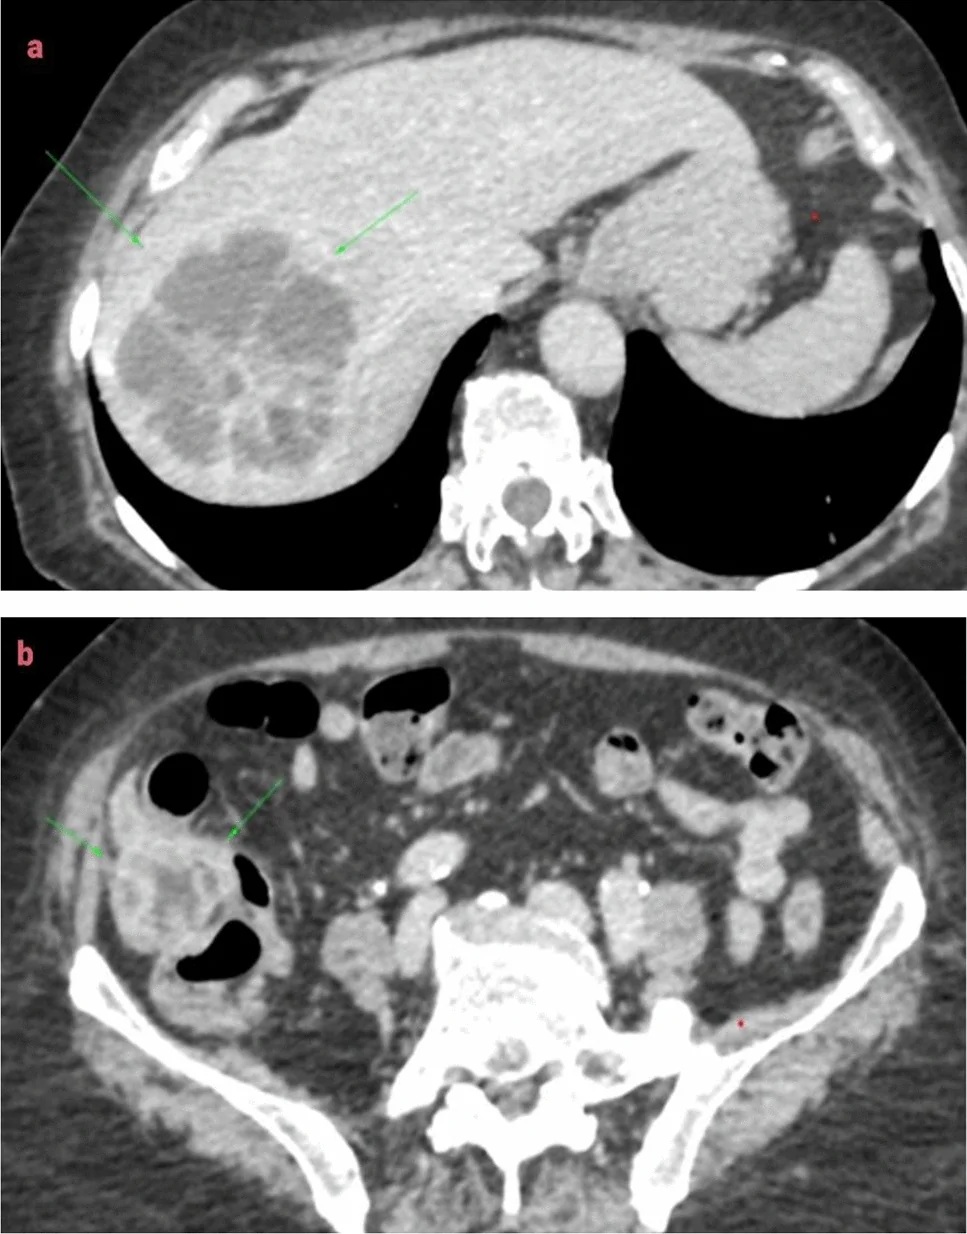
CT image of the liver (a) and appendiceal lesion (b)
